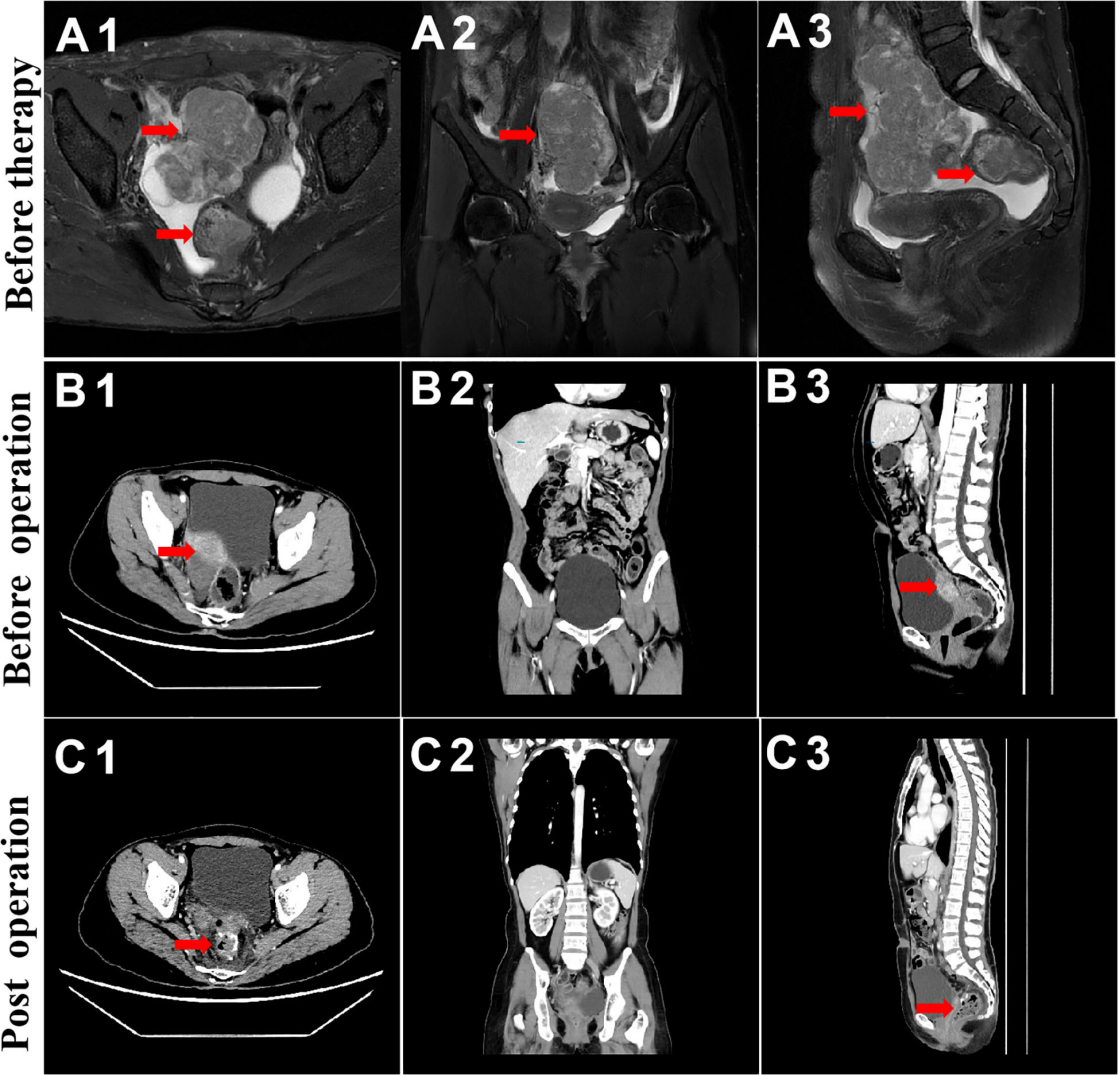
MRI and CT evaluation of ovarian and rectal tumors. From top to bottom, before chemotherapy and immunotherapy (A), before surgical treatment (B), and during 3-week postoperative follow-up (C).
